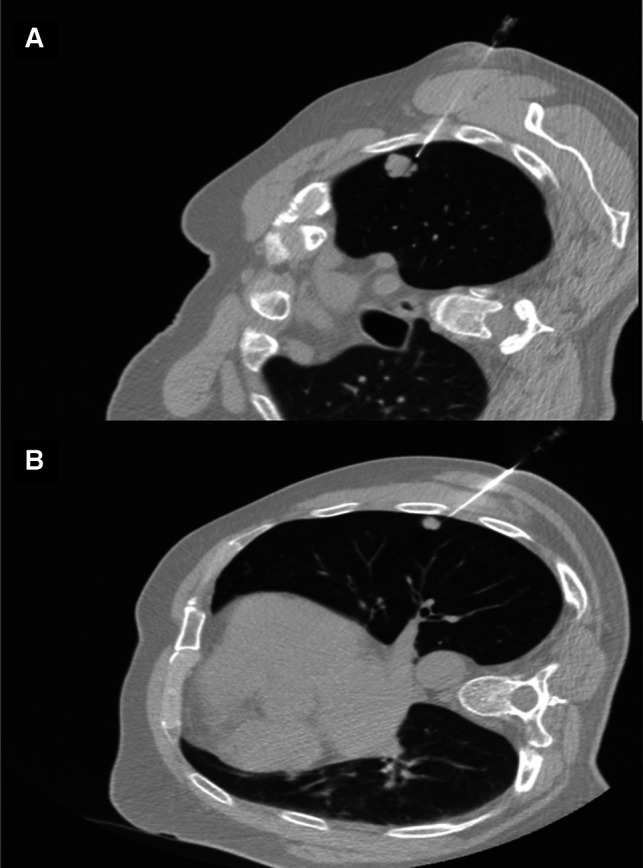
CT scan of needle injection of indocyanine green.
